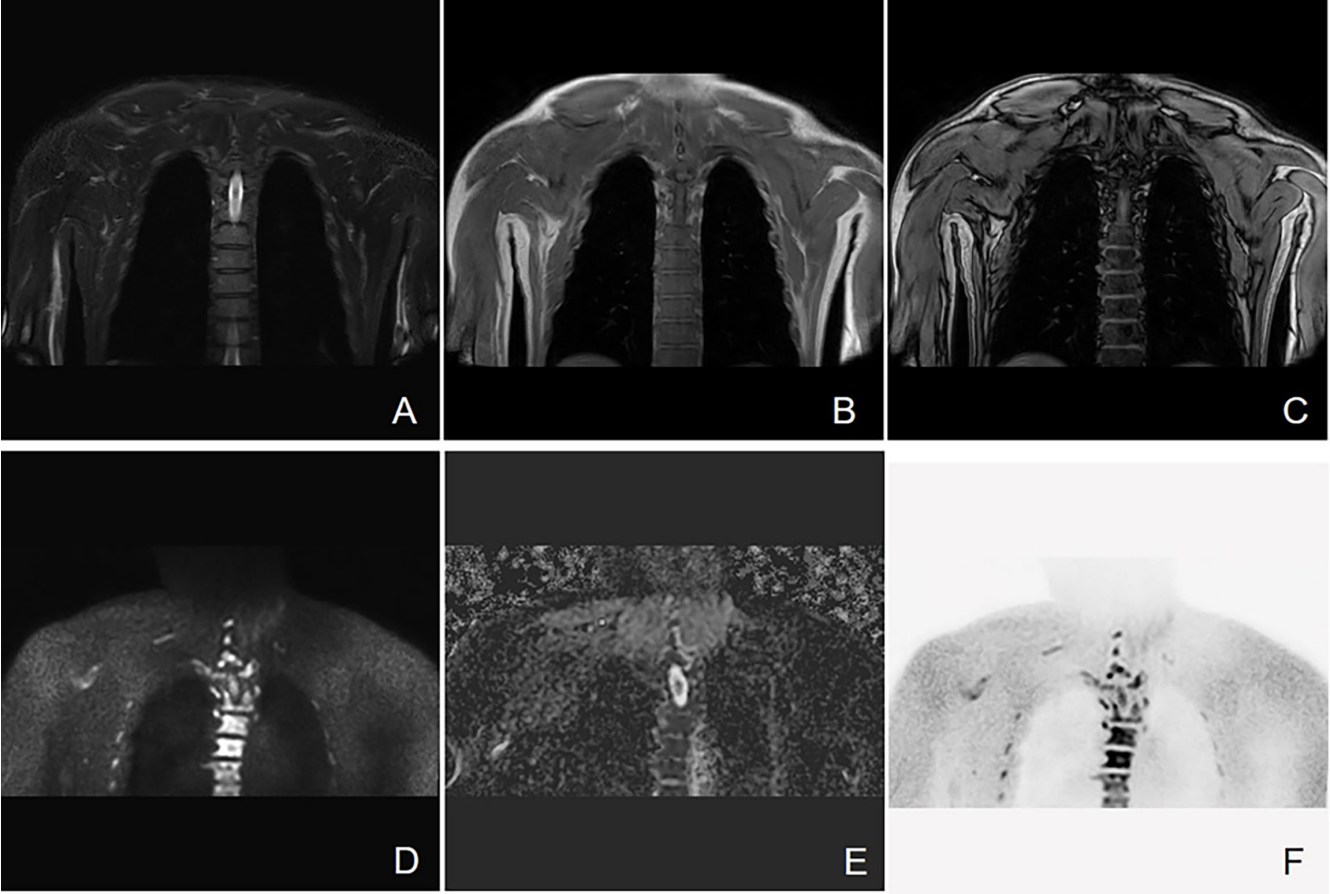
Active progressive MM disease. A case of a 63-year-old man who was diagnosed with MM (kappa light chain, RISS Stage III) is presented here. (A–C) Coronal images of T2 STIR, in-phase and out-phase of the chest. (D–F) Coronal images of DWI, ADC map, and inverted images, respectively. Diffuse abnormal signal is seen in the thoracic vertebrae, ribs, and right scapula within the scanning range, which show slightly low signal on T1WI and slightly high signal on T2 STIR. Significant high signal can be observed in DWI sequence.
